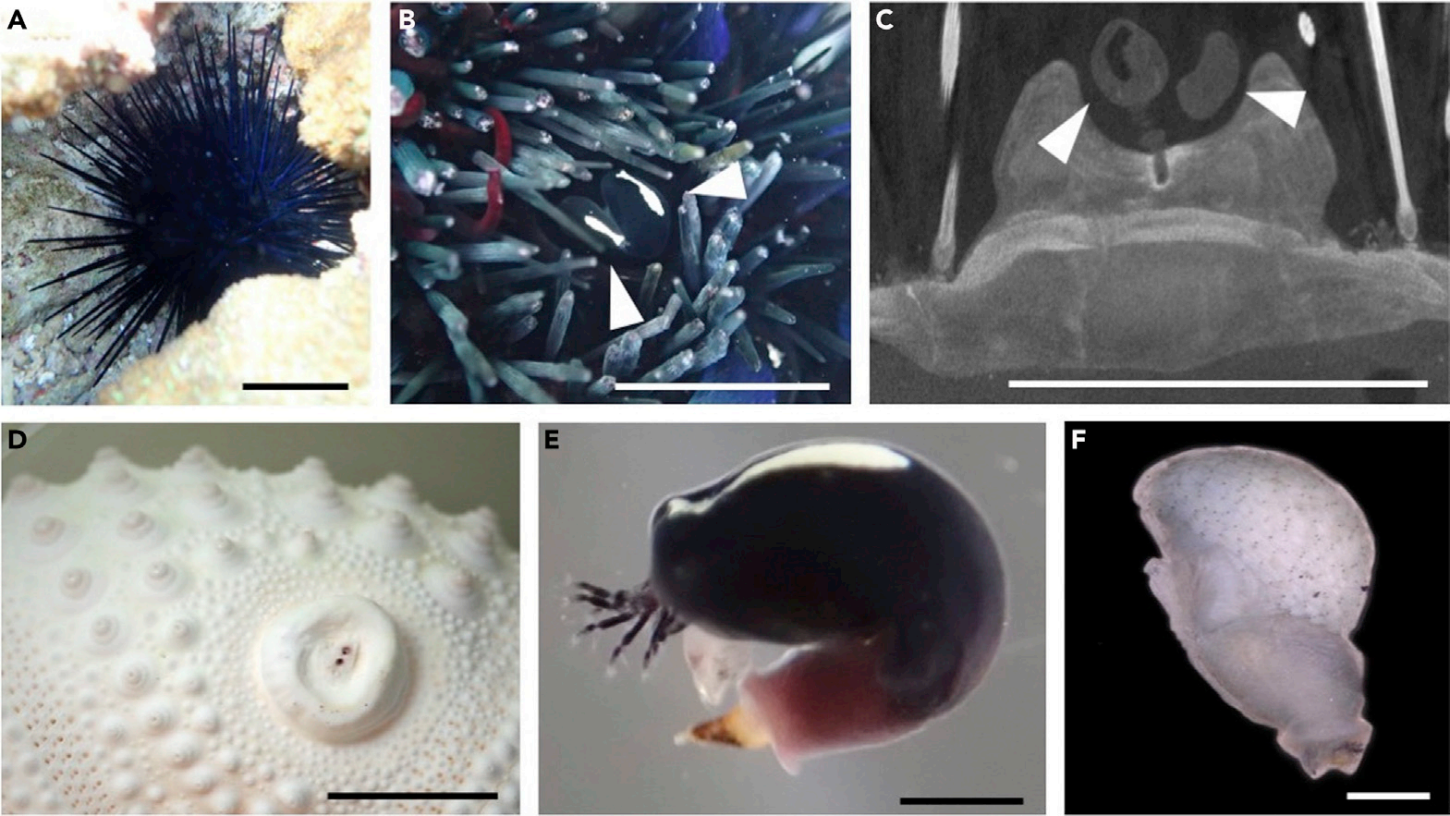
Habit, Morphology, and Phylogeny of *Rugilepas pearsei* (A) A living individual of *Echinothrix diadema* inhabiting crevice of coral reef. (B) Two living barnacles (arrowheads) attached to the base of a semi-open gall formed on the test of the host sea urchin *Echinothrix diadema*. (C) Computed tomography (CT) image of a gall inhabited by two barnacle individuals (arrowheads). (D) Undersurface of a bleached test of a galled sea urchin. (E) Lateral view of a detached individual with extended cirri. (F) Lateral view of a brooding individual after carapace removal. Scale bars: 10 cm in (A), 1.0 cm in (B)–(D), and 1.0 mm in (E) and (F). See also Figure S1 and Table S1.
